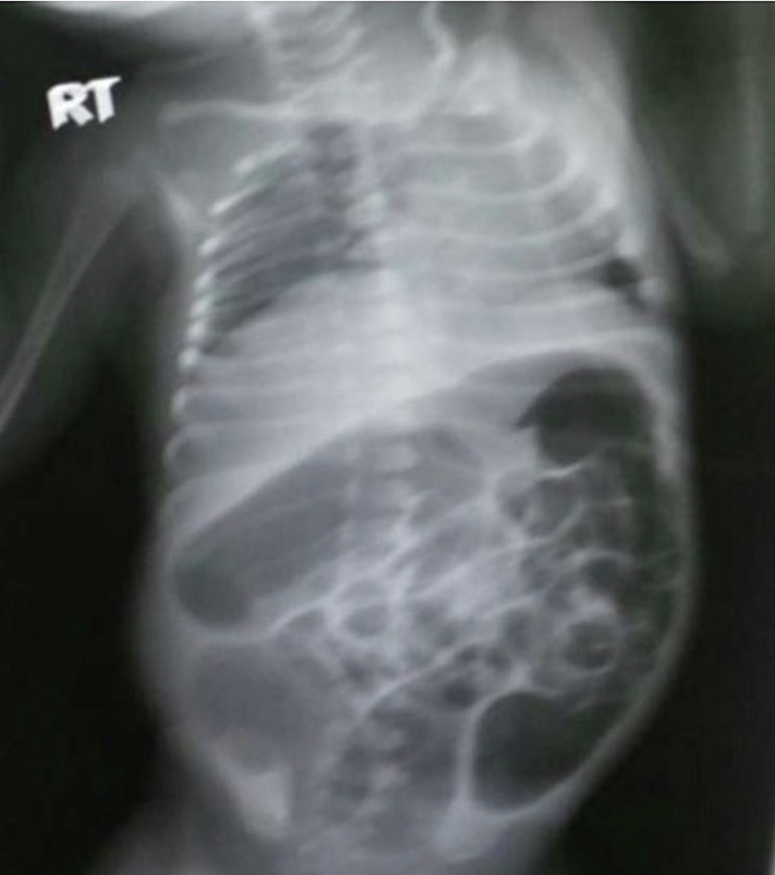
Anteroposterior abdominal x-ray revealed distended bowel loops with clearly defined air-fluid levels.
